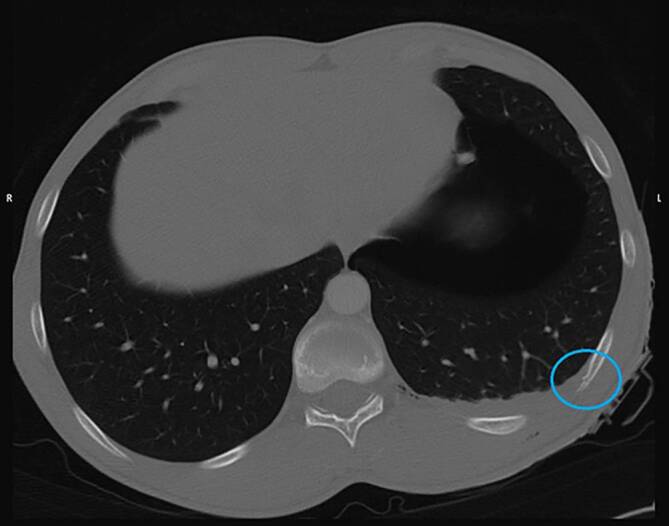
CT des Brustkorbs im Knochenfenster nach Messerstichverletzung links thorakal mit Fraktur der Rippe durch das Messer (*Kreis*)
